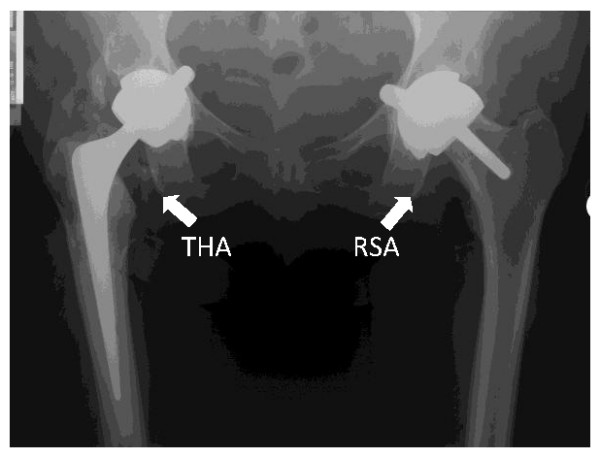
X-ray showing a Total Hip Arthroplasty (THA) and a Resurfacing Arthroplasty (RSA).
